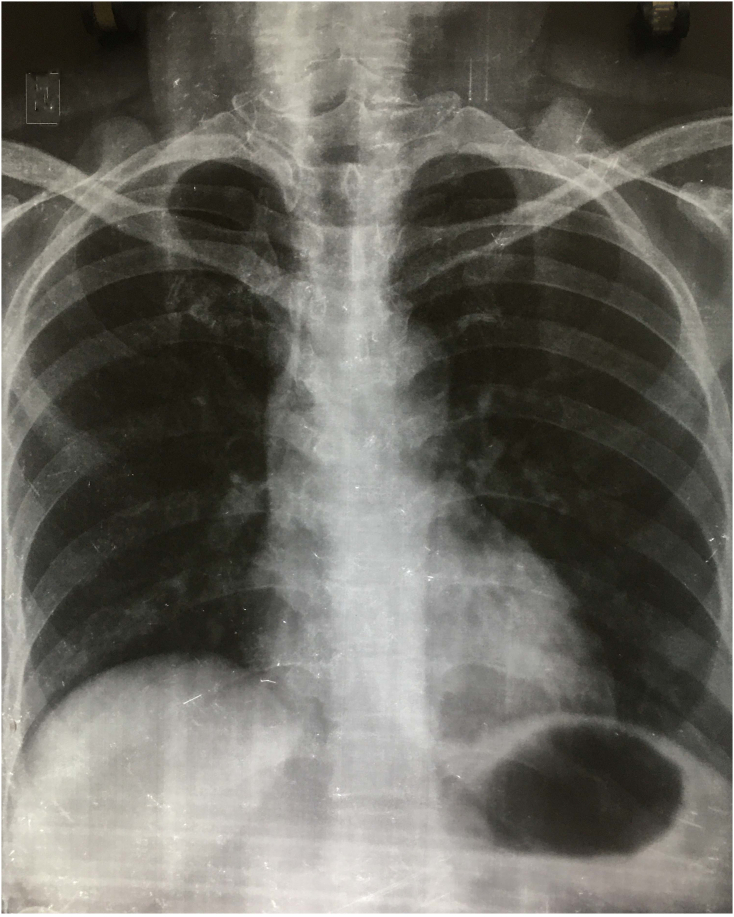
An erect X-ray chest showing no gas under right dome of diaphragm.
